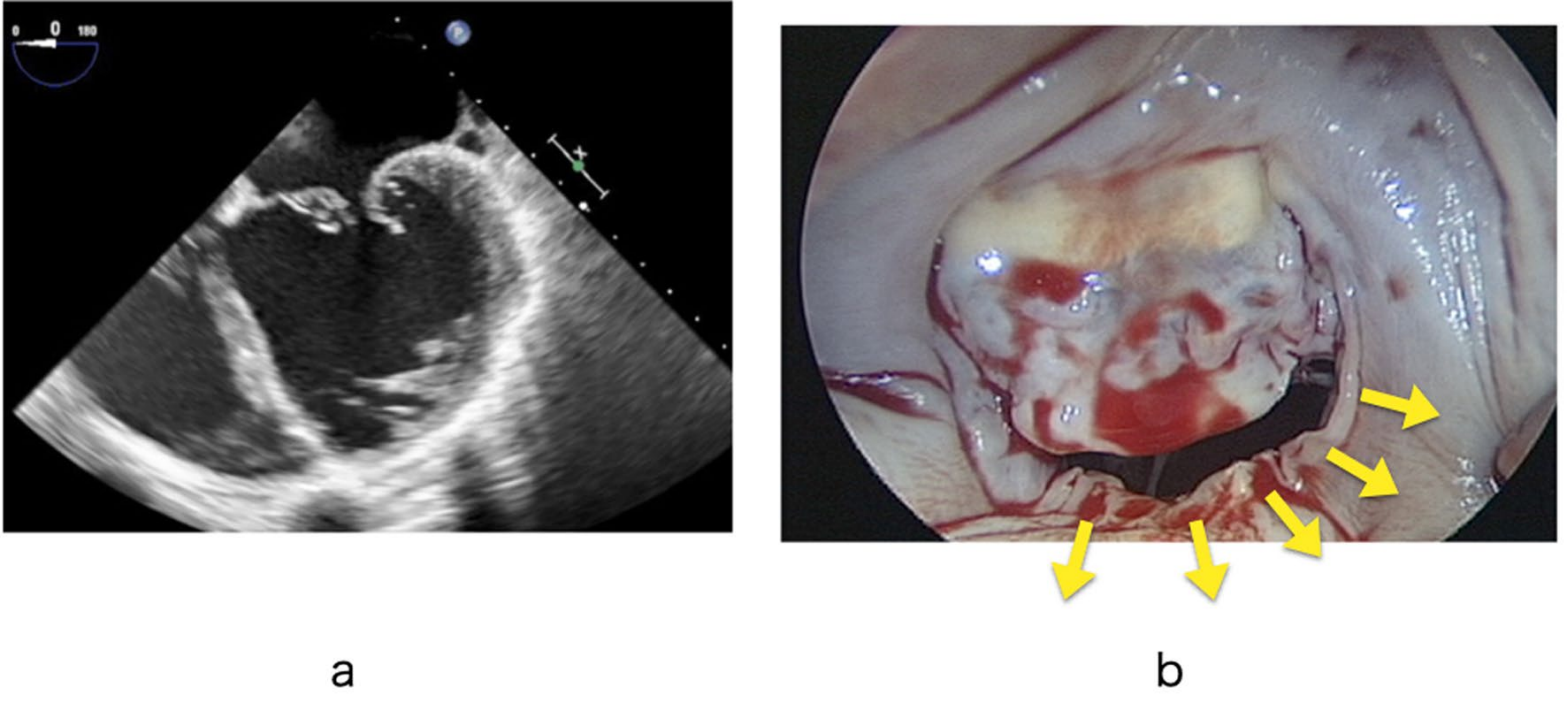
a Echocardiography of a patient with a giant left atrium showing a gap between the leaflets. b Photograph showing the large gap. The arrows show the direction of dilatation of the posterior annulus
